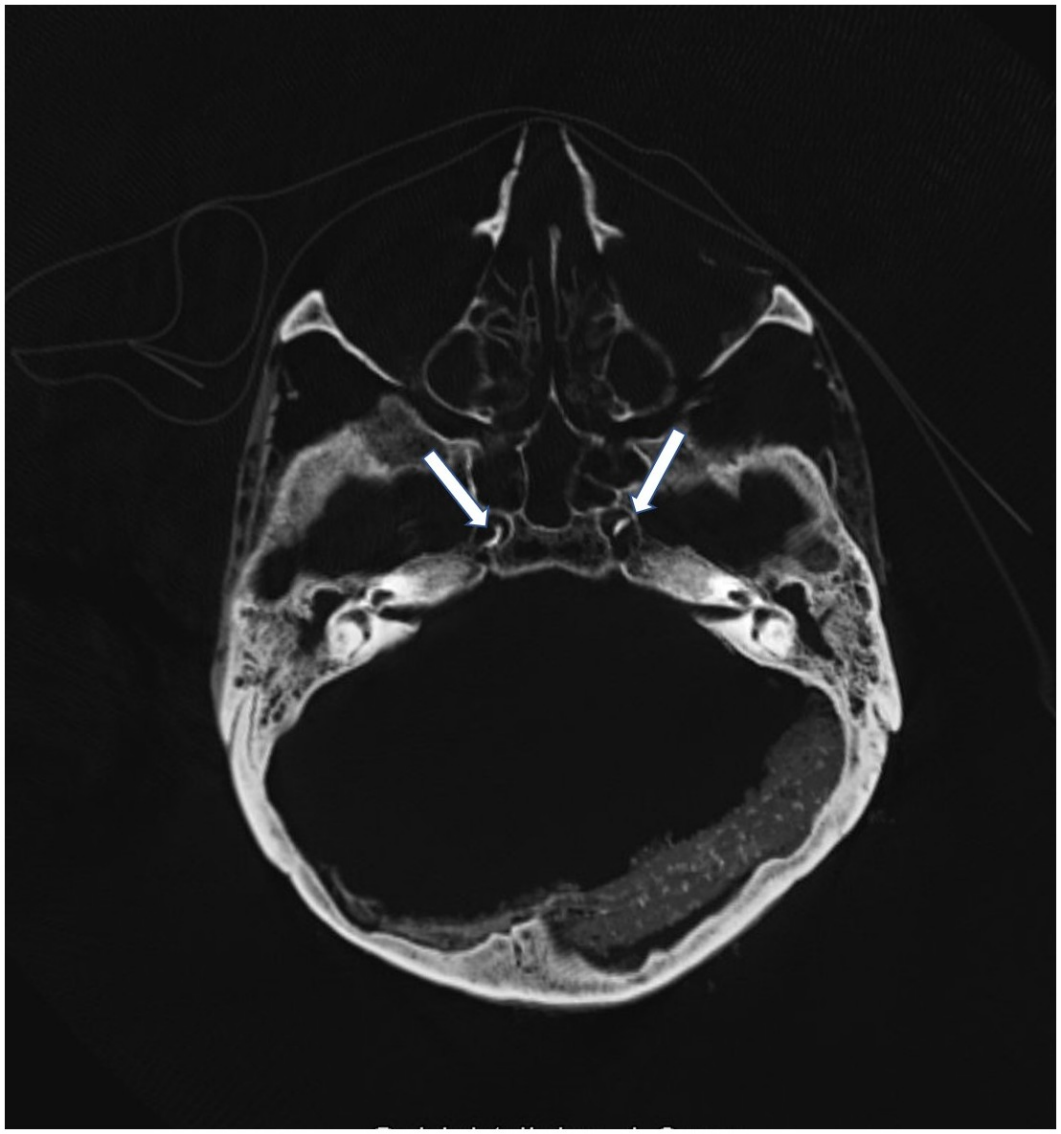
CT scan of the skull base showing bilateral calcifications of the internal carotid artery (arrows).
